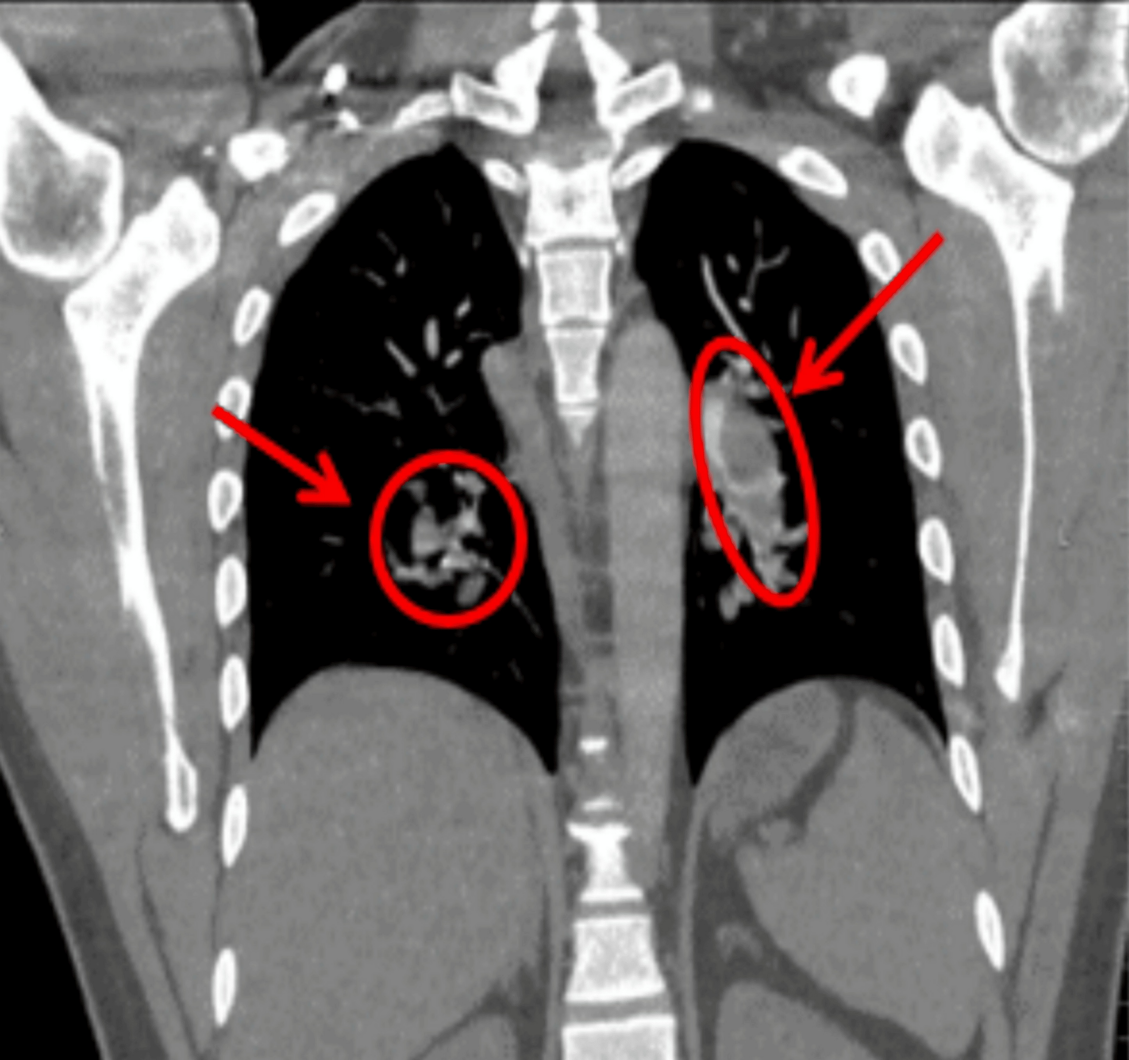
The CT pulmonary angiogram demonstrates filling defects within the right and left pulmonary arteries, extending into their branches and consistent with bilateral pulmonary emboli
